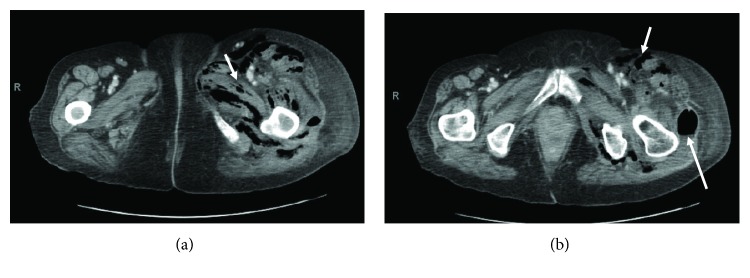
Axial CT images with IV contrast of the lower pelvis (a and b) demonstrating extensive subcutaneous emphysema consistent with necrotizing fasciitis around the left femur (short arrows). Note the air filled abscess cavity (b) filled along the posterior aspect of the left hip (long arrow).
